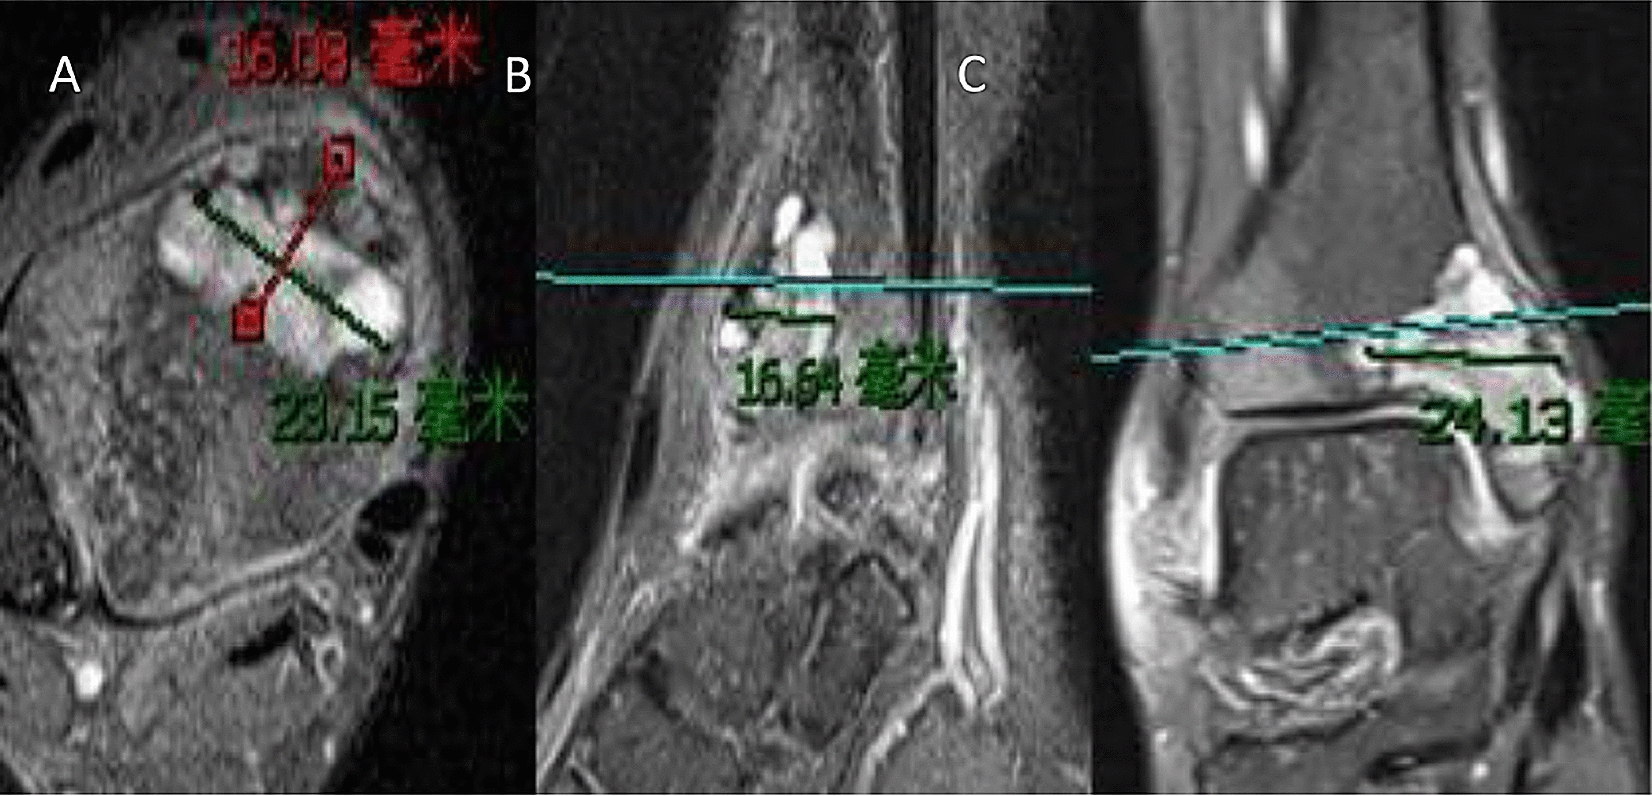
MRI plain scan of the ankle joint (October 20, 2017): a mass at the distal end of the right tibia, about 29 mm × 13 mm × 24 mm abnormal Constant signal, TIWL shows equisignal with clear margin and visible bone destruction, which is considered as giant cell tumor of bone (a–c)
